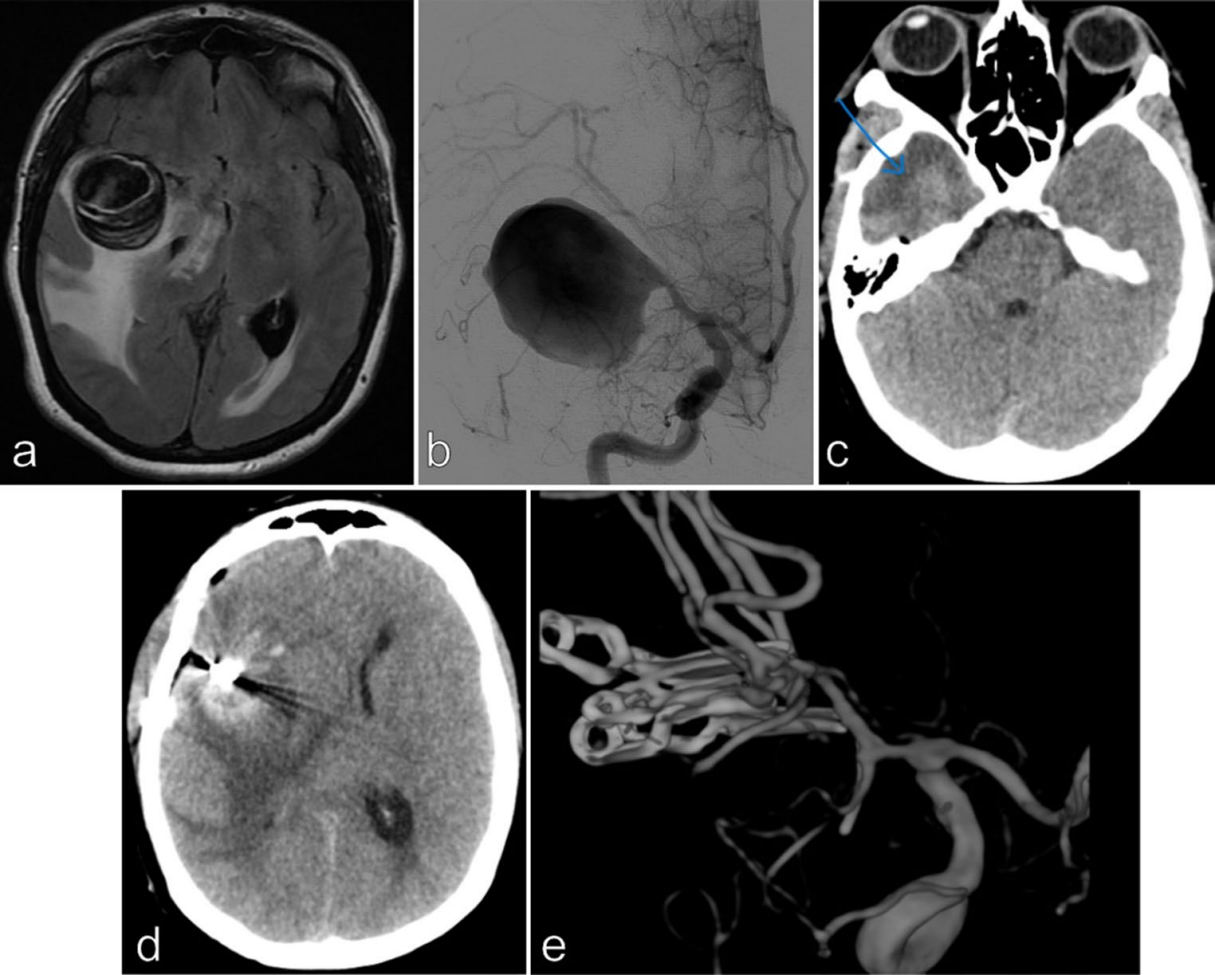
Patient 6, 44 yo female, without any medical history, complained about increasing headache for 1 month. CT scan, MRI/MRA and conventional angiography demonstrated a right giant MCA aneurysm (maximal diameter = 37 mm). A significant peri-aneurysmal edema and intrasaccular thrombus were noted. The midline brain shift was 10 mm (a and b). Deep hypothermic circulatory arrest/extra-corporeal circulation with vascular reconstruction was performed to exclude the aneurysm from the circulation. A deliberate partial temporal lobe resection (blue arrow) was performed at the beginning of the surgical procedure to gain a better exposure of the aneurysm (c and d). The patient was extubated after 48 h without any neurological deficit. Complete occlusion of the aneurysm was noted (e). No neurological sequelae were noted and the mRS score was 1 after 3 months
